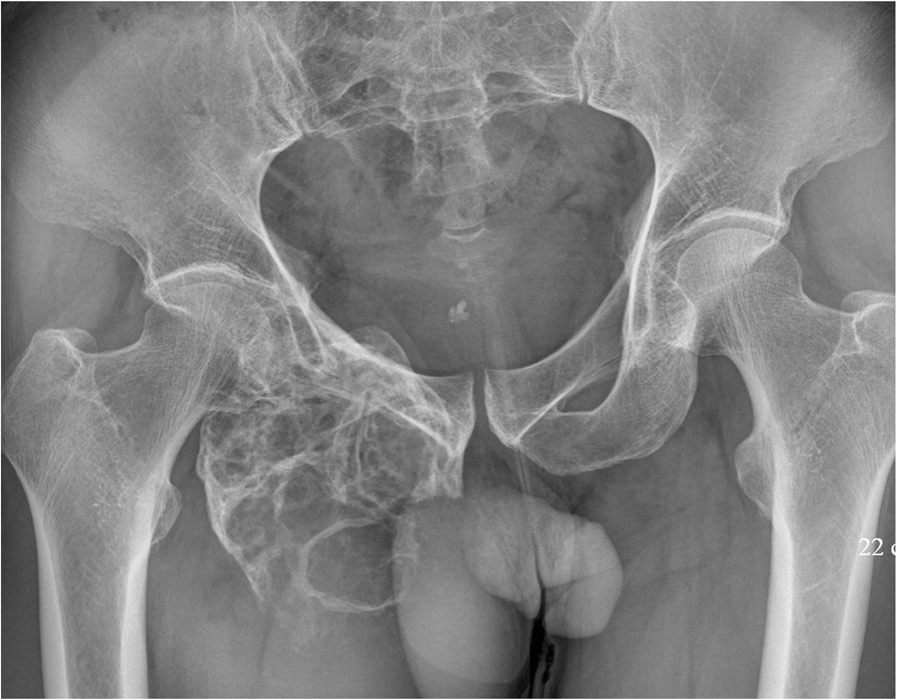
LGMS of the right shoulder blade. Pre-operational CT scan revealed an irregular mass with massive calcification and ossification, and pulmonary metastasis
